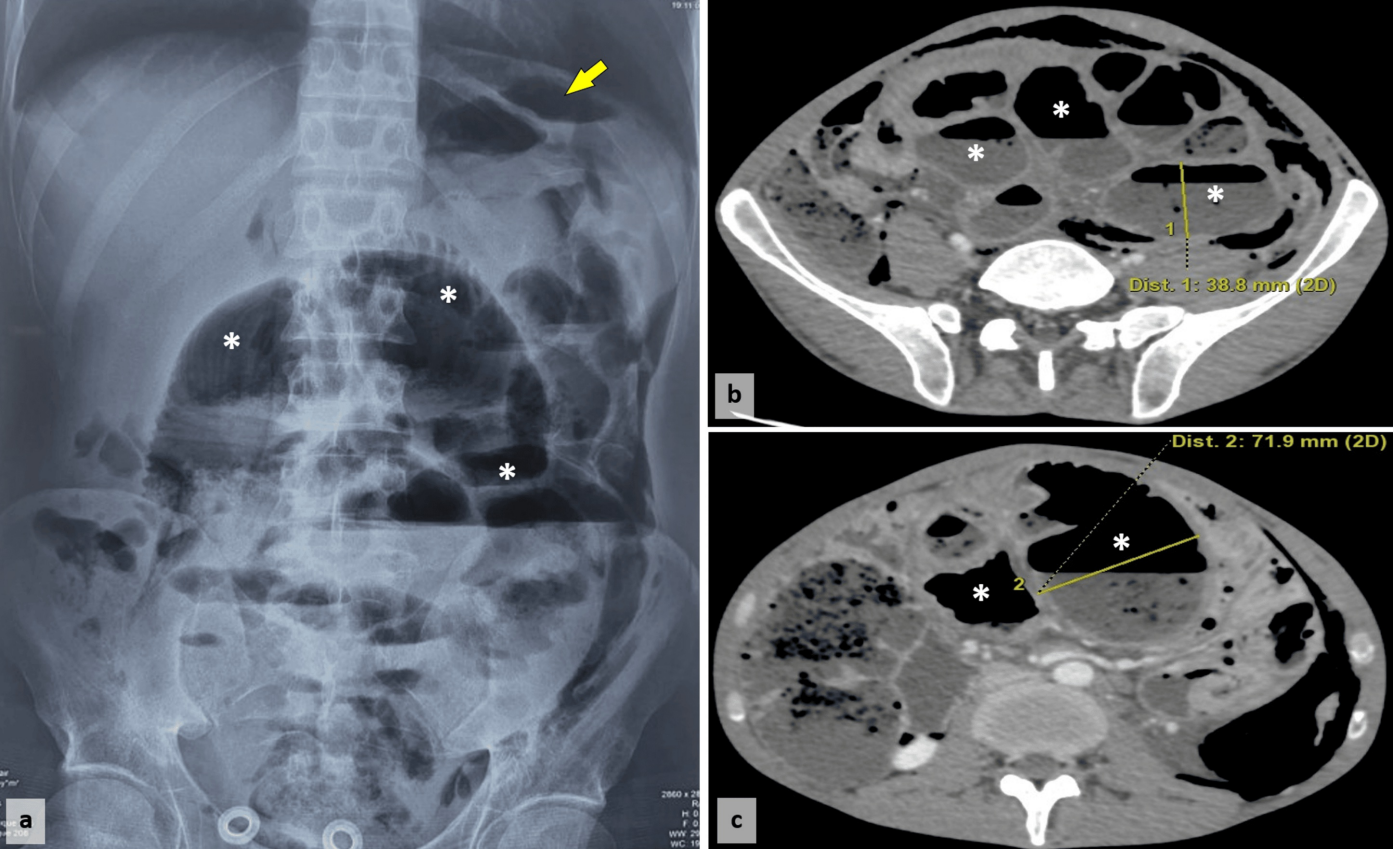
Abdominal X-ray showing marked gaseous distension of bowel loops (*), suggestive of bowel obstruction, along with pneumoperitoneum (arrows) (a). Axial abdominal CT scan showing multiple air-fluid levels and distended intestinal loops (*) (b and c).
